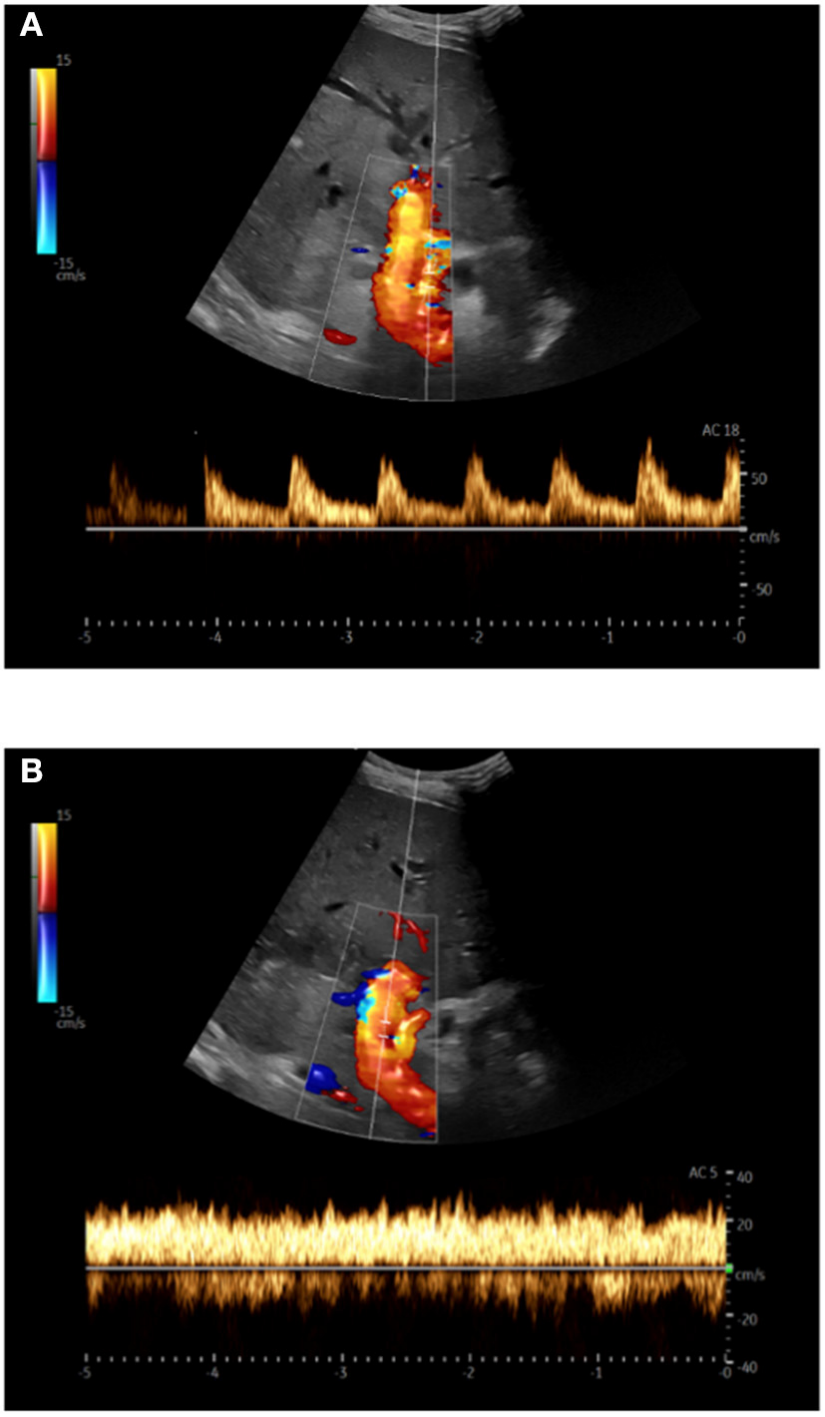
Doppler sonography in triplex technique (B-image + color Doppler + spectral Doppler) in a patient with acute-on-chronic liver failure; (A) Hepatic artery: Derivation of the arterial flow signal; (B) Portal vein: The maximum flow velocity was measured at the level of the hepatic artery after angle correction.
